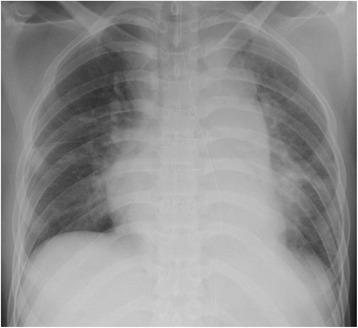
Chest X-ray showed a wide mediastinum, enlarged aortic knob, rightward deviation of the nasogastric tube, and a smudgy aortopulmonary window
